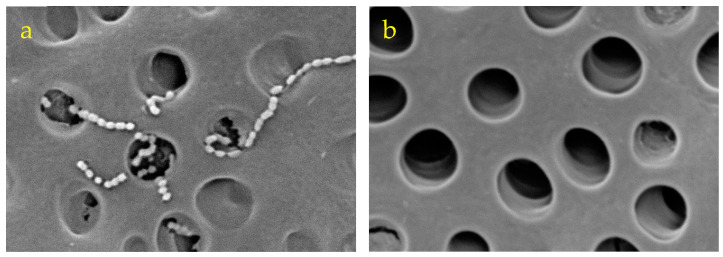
SEM images before (a) and after (b) treatment for bacterial detachment. *S. sobrinus* that entered the dentin tubular canal opening were completely removed with ultrasonic cleaning. Magnification: ×5000.
